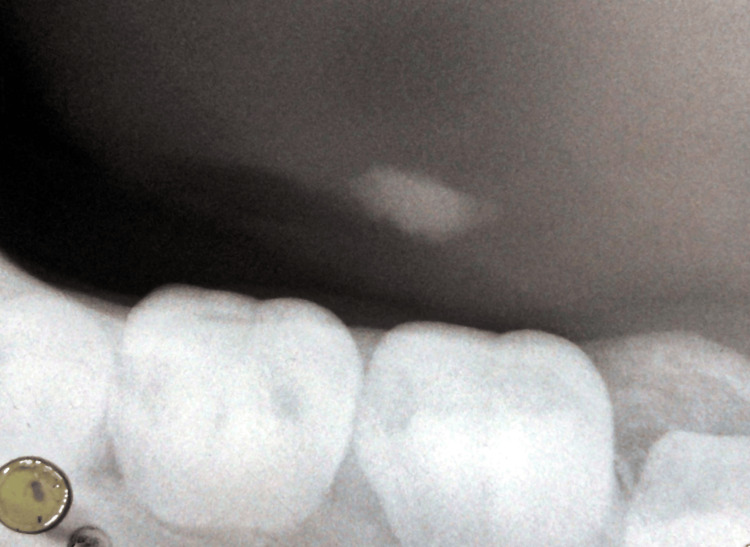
A mandibular occlusal radiograph shows radiopacity medial to teeth numbers 36 and 37.
